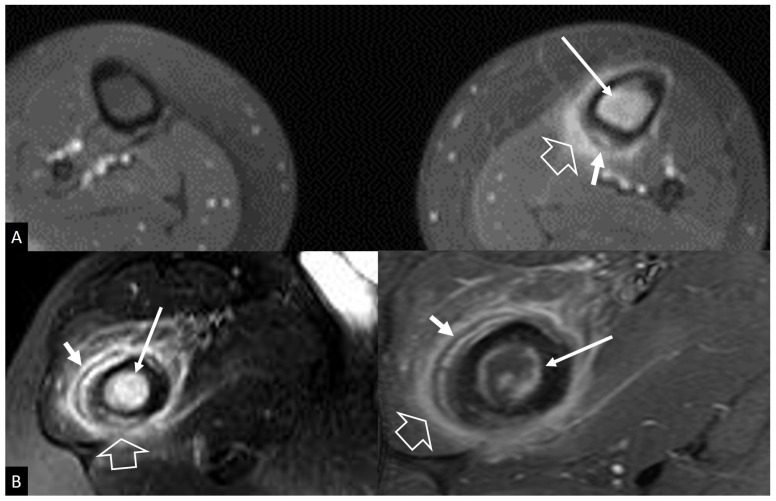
(A) Stress reaction in an 11-year-old boy with pain in the tibia exaggerated with football training. Axial fat-suppressed contrast-enhanced T1-w MR image, showing the bone marrow edema (long thin arrow), the soft-tissue edema (open arrow), and the periosteal reaction (short arrow). (B) Ewing’s sarcoma in the right proximal femoral bone in a 17-year-old male patient. Axial fat-suppressed T2-w (left) and fat-suppressed contrast-enhanced T1-w (right) MR images showing the bone marrow edema (long thin arrow), the soft-tissue edema (open arrow), and the multilayered periosteal reaction (short arrow).
